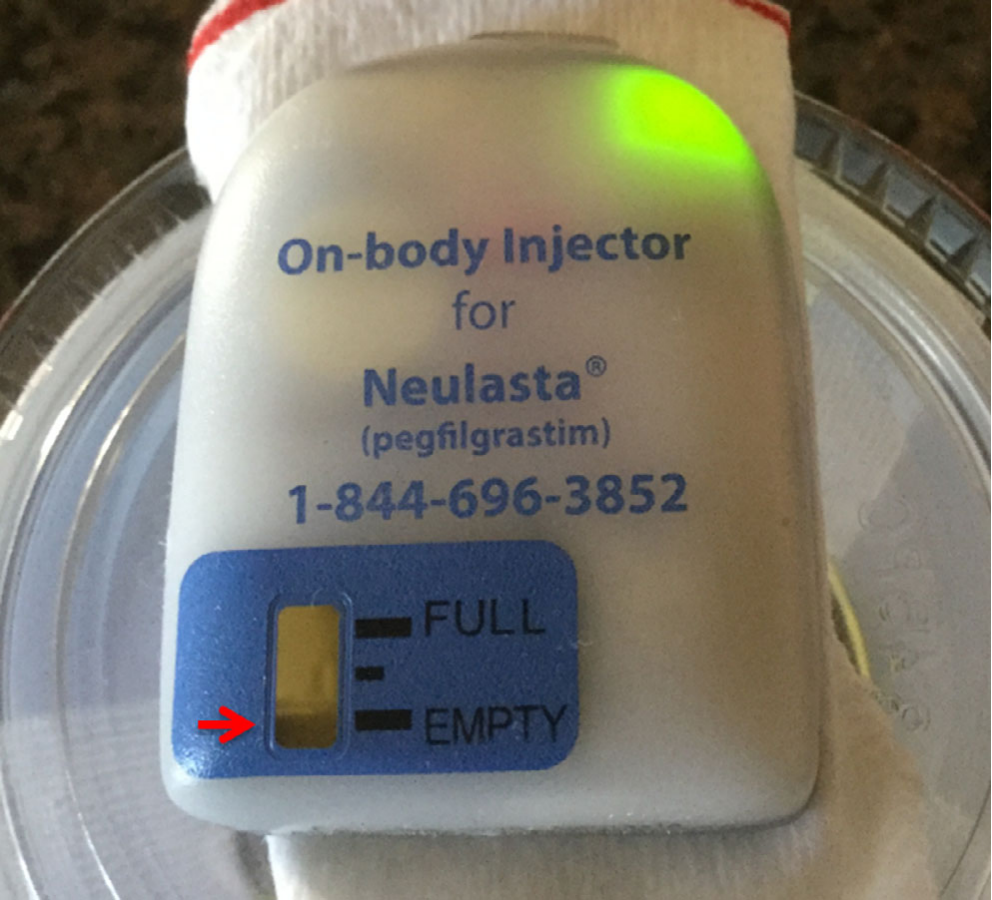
Photograph of the device at the end of drug delivery after ten computed tomography scans with a cumulative dose of 230 mGy CTDIvol. As expected, the indicator read empty (arrow), and the status light flashed green.
